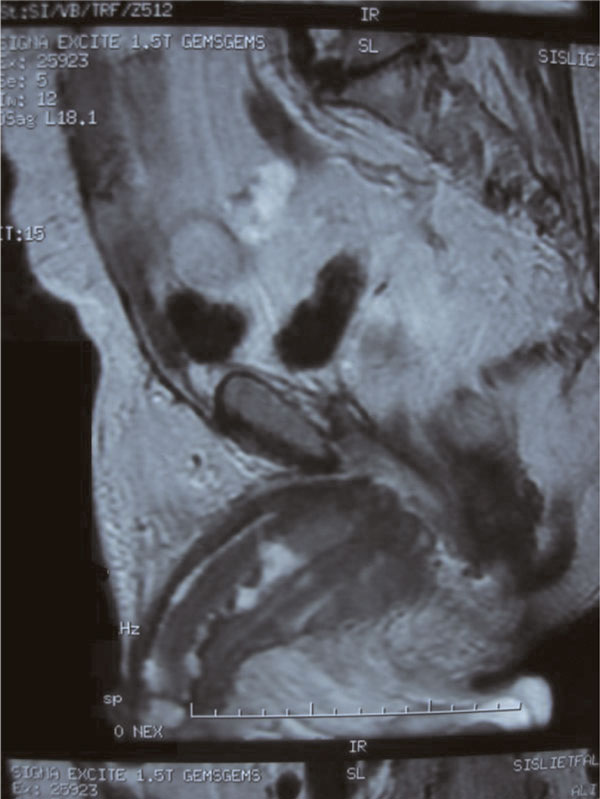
Infiltration of cavernosal bodies on MRI.
